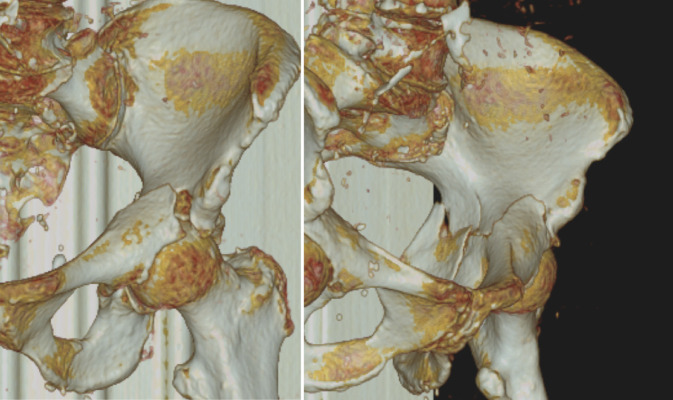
In der präoperativen CT-Diagnostik kann die genaue Frakturmorphologie festgestellt und mögliche Therapieoptionen können eruiert werden. In der Rekonstruktion stellt sich die leicht dislozierte T‑Fraktur des Azetabulums Typ 6 nach Letournel und Judet dar
